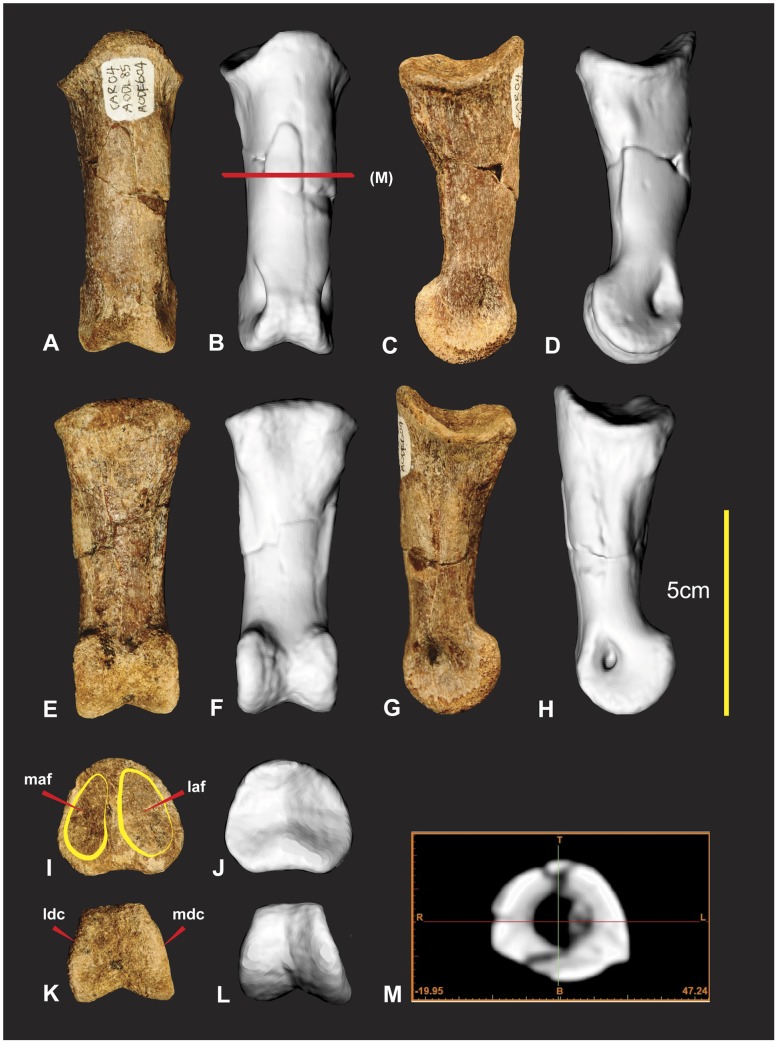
Right manual phalanx III-1. Right manual phalanx III-1 in dorsal (A & B), lateral (C & D), ventral (E & F), proximal (G & H), mid shaft CT image (M). *Abbreviations:* laf, lateral articular facet; ldc, lateral distal condyle; maf, medial articular facet; mdc, medial distal condyle.
